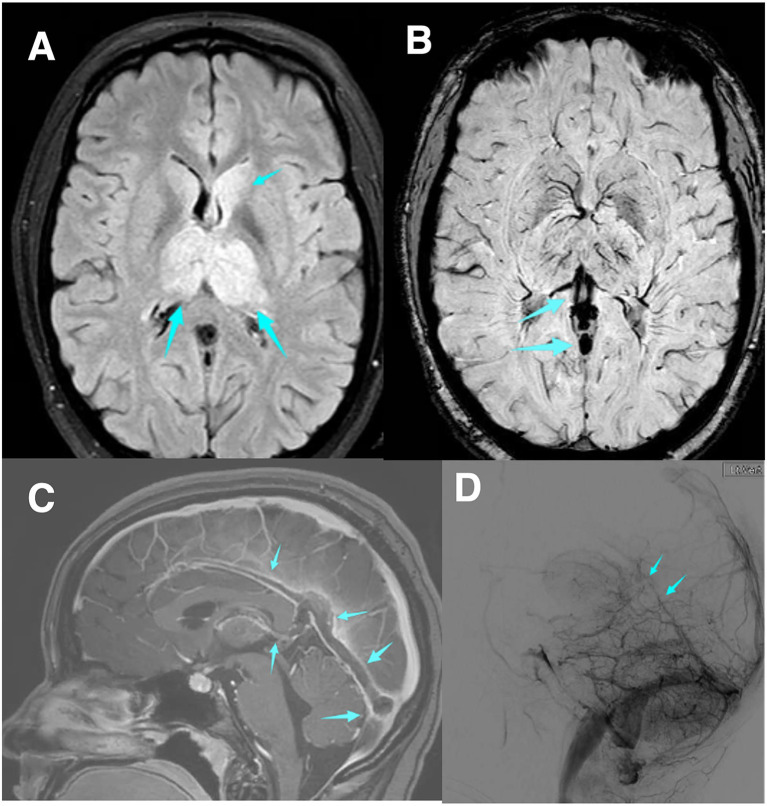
Deep cerebral venous thrombosis with multimodal imaging findings. (A) Axial FLAIR demonstrating bilateral thalamic hyperintensity with associated edema extending into the caudate nucleus, as indicated by arrows. (B) Susceptibility-weighted imaging showing dilation of the deep venous system consistent with thrombosis. (C) Sagittal T1-weighted post-contrast imaging demonstrating absence of enhancement in the vein of Galen, inferior sagittal sinus, and straight sinus. (D) Catheter angiography demonstrating impaired deep venous outflow consistent with thrombosis.
